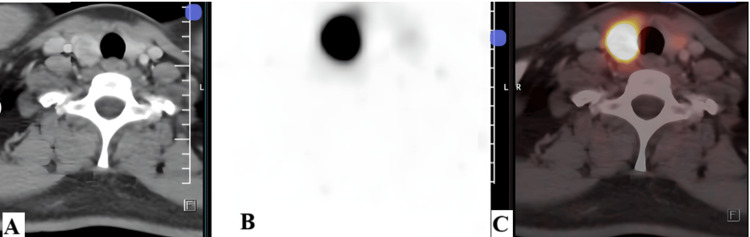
Ga-68-DOTANOC PET/CT demonstrating DOTANOC-avid thyroid nodule (A) Axial contrast-enhanced CT image at the level of the thyroid gland demonstrating a well-defined, arterially heterogeneously enhancing nodule in the right lobe of the thyroid. The left thyroid lobe appears grossly unremarkable. (B) Axial PET image showing focal increased radiotracer uptake corresponding to the right thyroid lobe lesion. (C) Axial fused PET/CT image demonstrating intense DOTANOC avidity within the right thyroid nodule (SUVmax 67.8), suggestive of significant somatostatin receptor expression SUV: standardized uptake value
